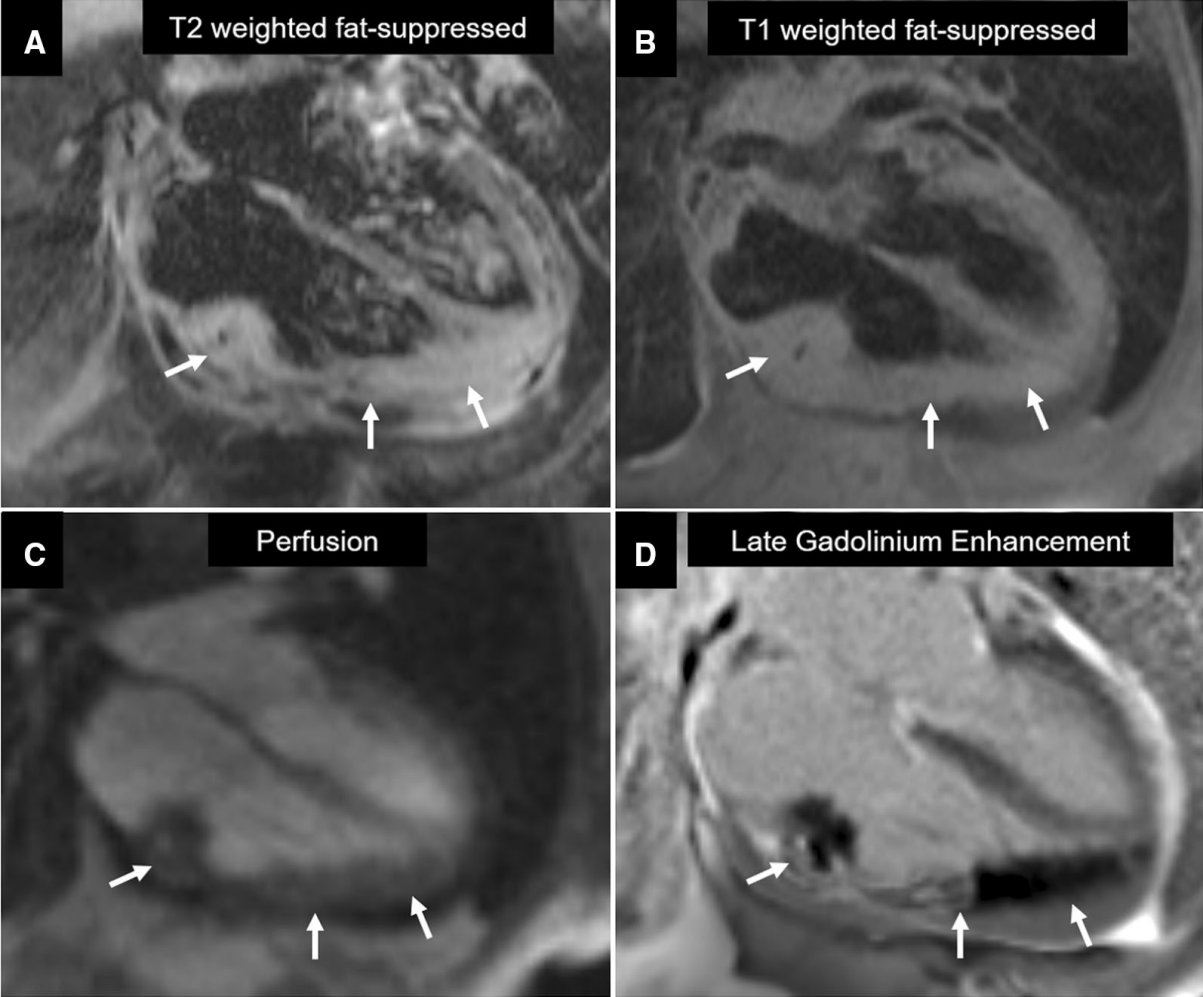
Baseline CMR tissue characterization and perfusion of the mass. A T2-weighted fast-suppressed imaging showing hyperenhancement of the mass, compatible with edema. B T1-weighted fat-suppressed sequence showing isointensity of the heterogeneous mass. C First-pass perfusion images show diffuse but homogeneous enhancement of the tumor. D T1-weighted inversion recovery showing heterogeneous late gadolinium enhancement of the mass
